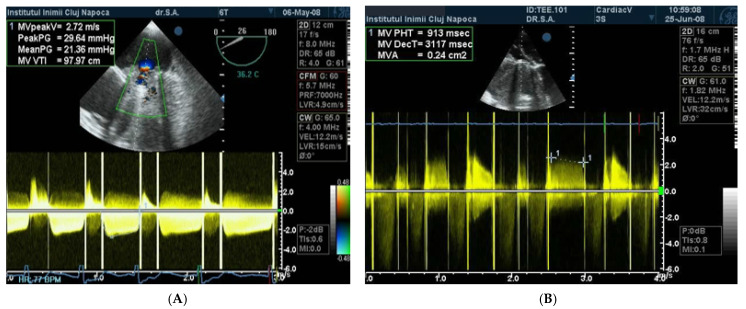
(A) TTE CW Doppler. Obstruction of mitral prosthesis PHT > 200 ms. (B) TEE CW Doppler. High gradients and velocities on mitral mechanical prosthesis are suggestive for obstruction.
